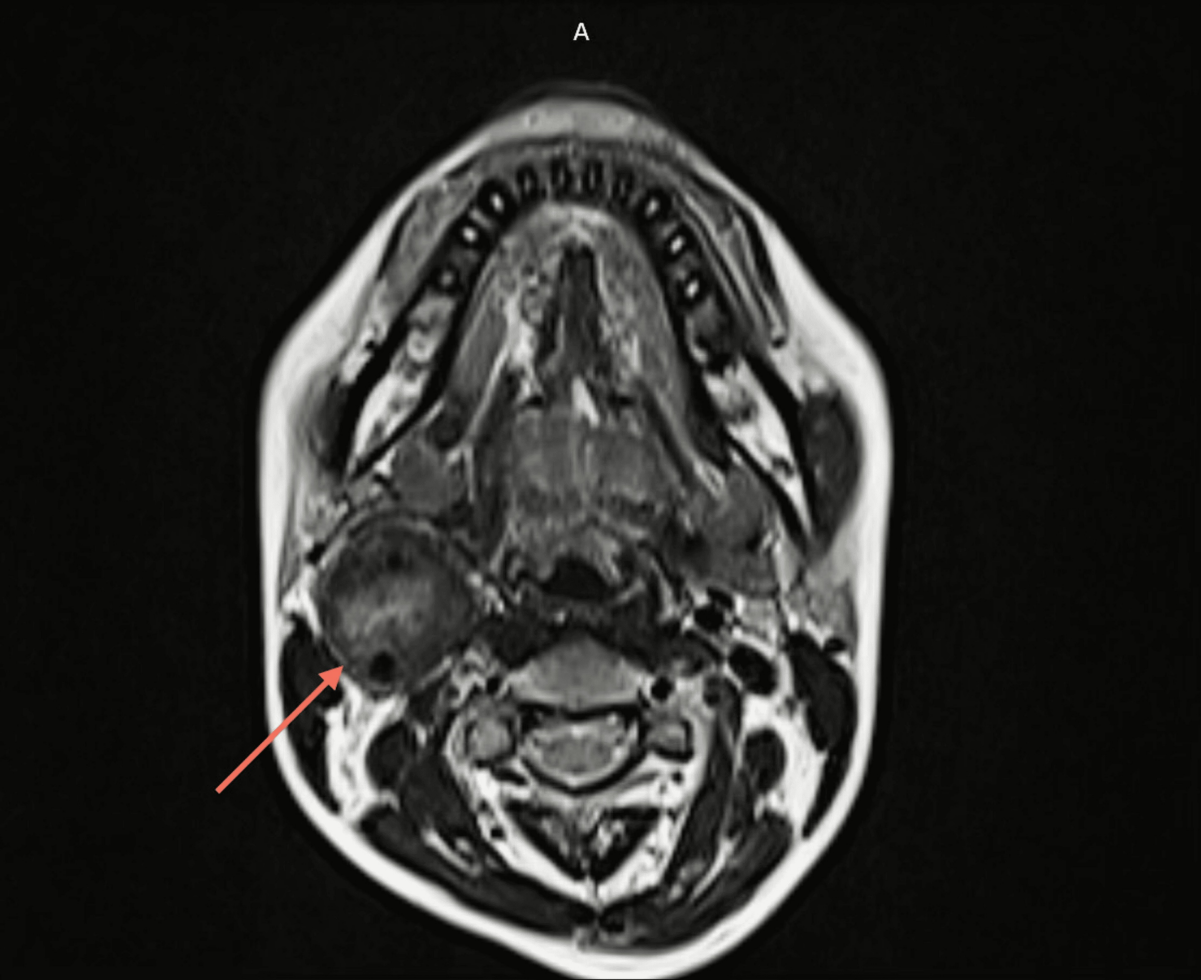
T1-weighted axial section of the magnetic resonance angiography of the head and neck region. The arrow depicts the tumor, with the characteristic salt-and-pepper appearance, on the right side.
